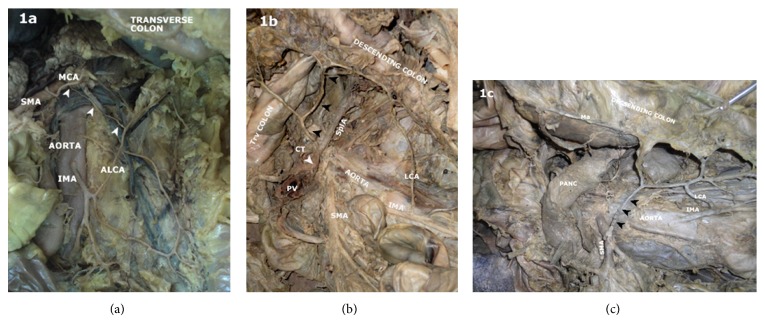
Photograph of the posterior abdominal wall showing the aorta and inferior mesenteric artery (IMA) and its branches. (a) A thin arterial channel (arrowheads) connecting middle colic (MCA) branch of superior mesenteric artery (SMA) to the ascending branch of left colic artery (ALCA) is observed. (b) Arrowheads show an arterial connection between the celiac artery (CT) and the left colic artery (LCA) ascending branch. SplA: splenic artery, PV: portal vein, Trv colon: transverse colon. (c) An inner arterial channel (arrowheads) besides the marginal artery (Ma) connecting the proximal SMA to LCA. PANC: pancreas.
